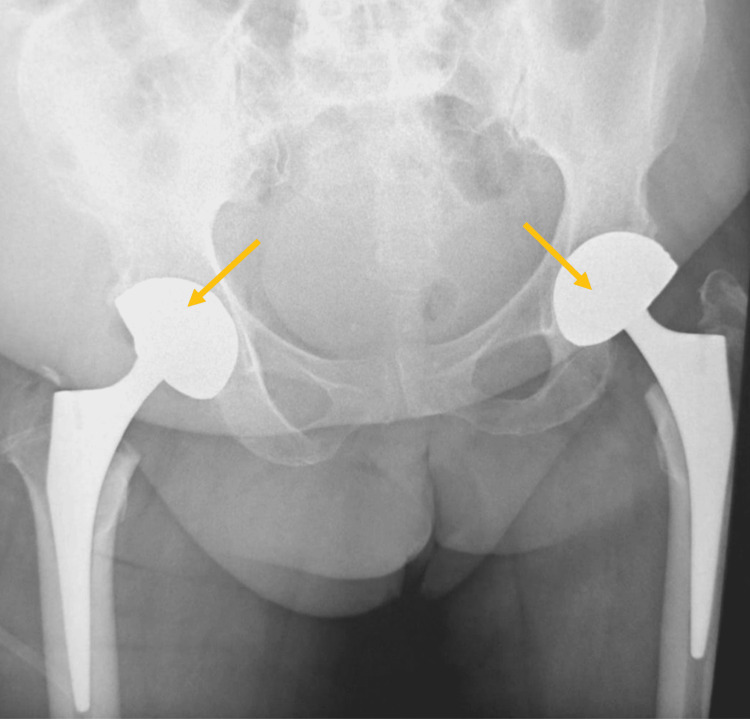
Postoperative plain radiography after a total hip replacement.
